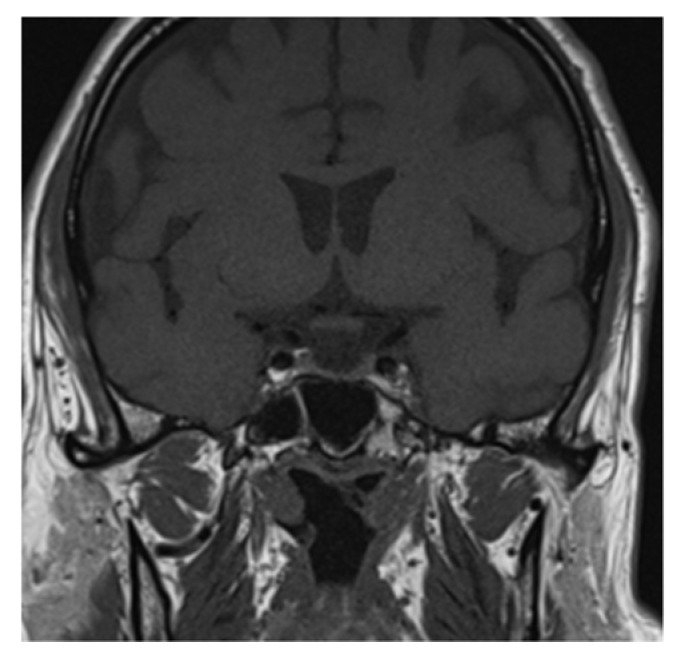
Partial “empty sellae syndrome” on brain MRI sagittal section. Pituitary gland reduced in size and lying along the floor of sphenoid sellae.
